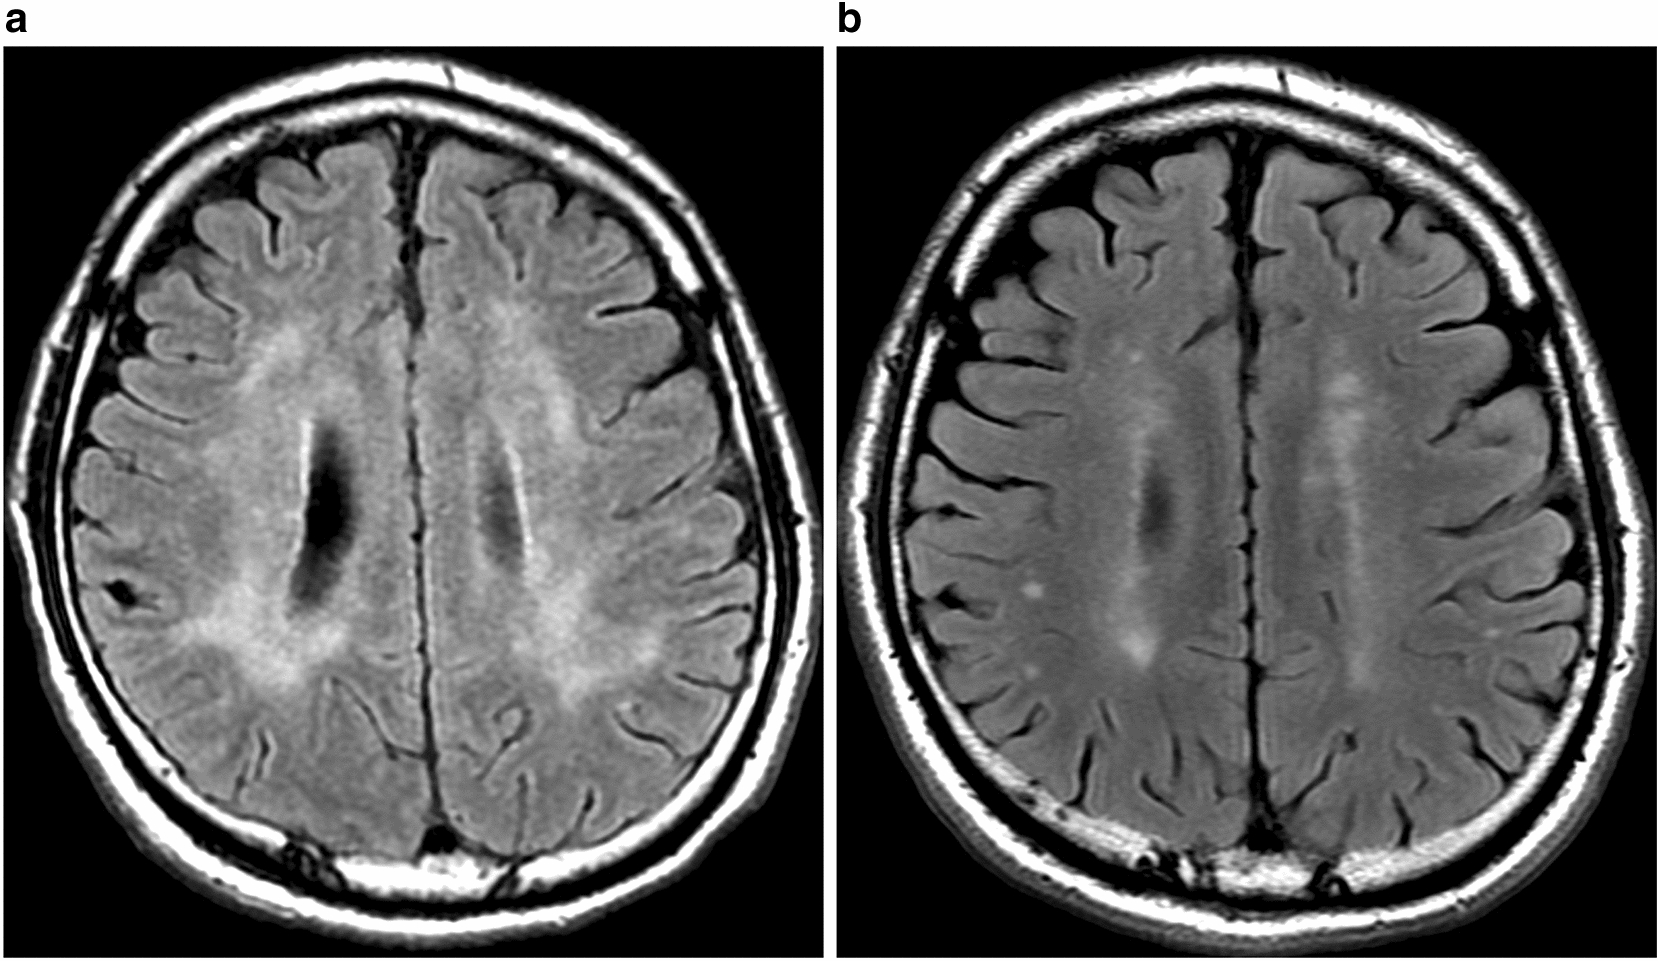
Axial FLAIR brain MR image on admission (a) and post-ART (b). a Symmetrical and diffuse cortical and central atrophies and an extensive high signal of the white matter were detected. b A decrease in white matter signals compared to pre-ART
